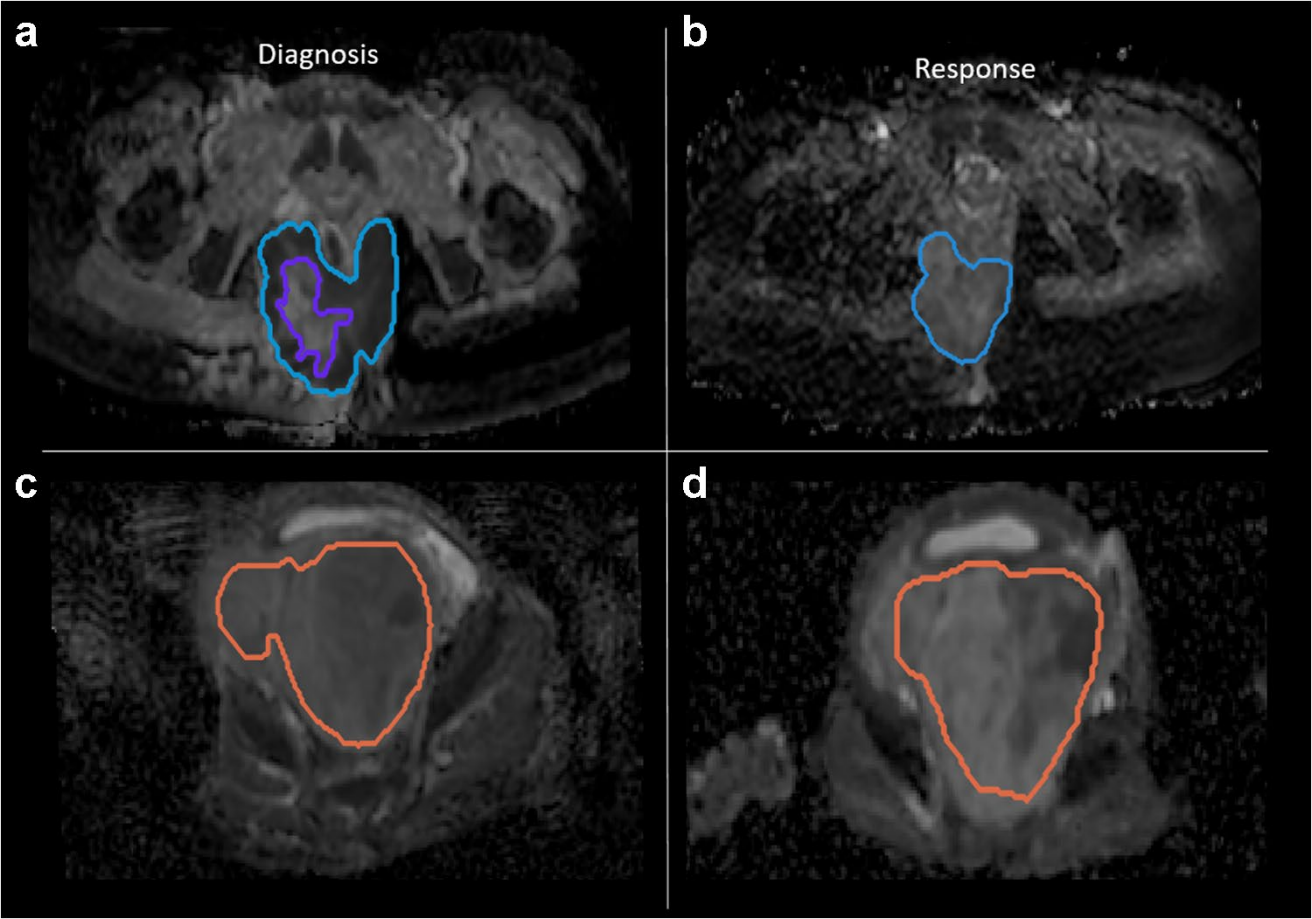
Tumor segmentation of diagnosis (a, c) and response (b, d) axial diffusion-weighted MRI (apparent diffusion coefficient) scans. a, b A 16-year-old girl with a perianal alveolar rhabdomyosarcoma. The whole tumor (*blue outline*) is delineated. The hemorrhagic component (*inner purple outline* in a) was excluded from the analysis. c, d A 1-year-old boy with a retroperitoneal pelvic rhabdomyosarcoma. The whole tumor (*red outline*) is delineated
